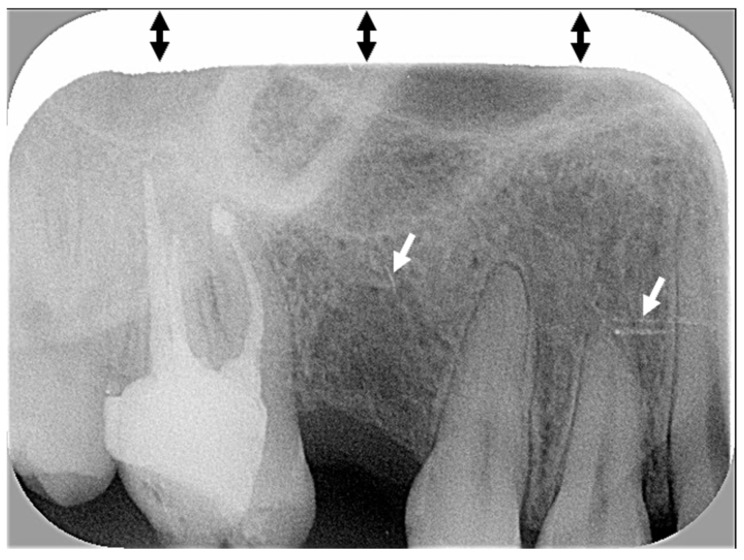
A truncated X-ray due to poor calibration of the digital plate scanner (black double arrows). Scratches are shown with white arrows.
